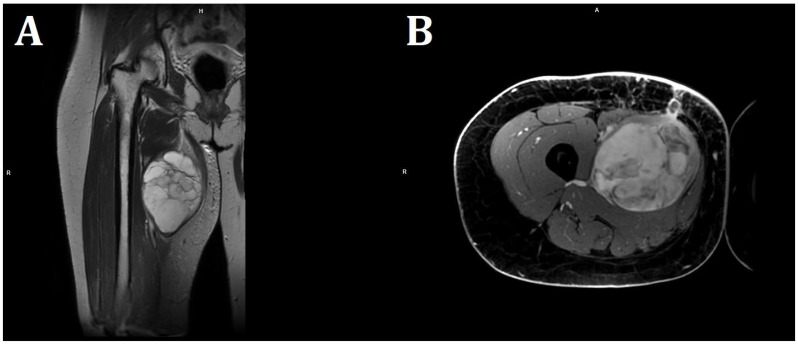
MRI imaging of a massive right thigh tumor. (A) shows a coronal T2-weighted image, and (B) shows an axial contrast-enhanced T1-weighted image. The signal intensity of the tumor is very bright on the T2-weighted image (A), suggesting that the tumor contains myxoid parts. The contrast-enhanced T1-weighted image shows a strong contrast enhancement of the tumor (B) (Philips MR 7700, 3.0 T; Philips, Cambridge, USA).
